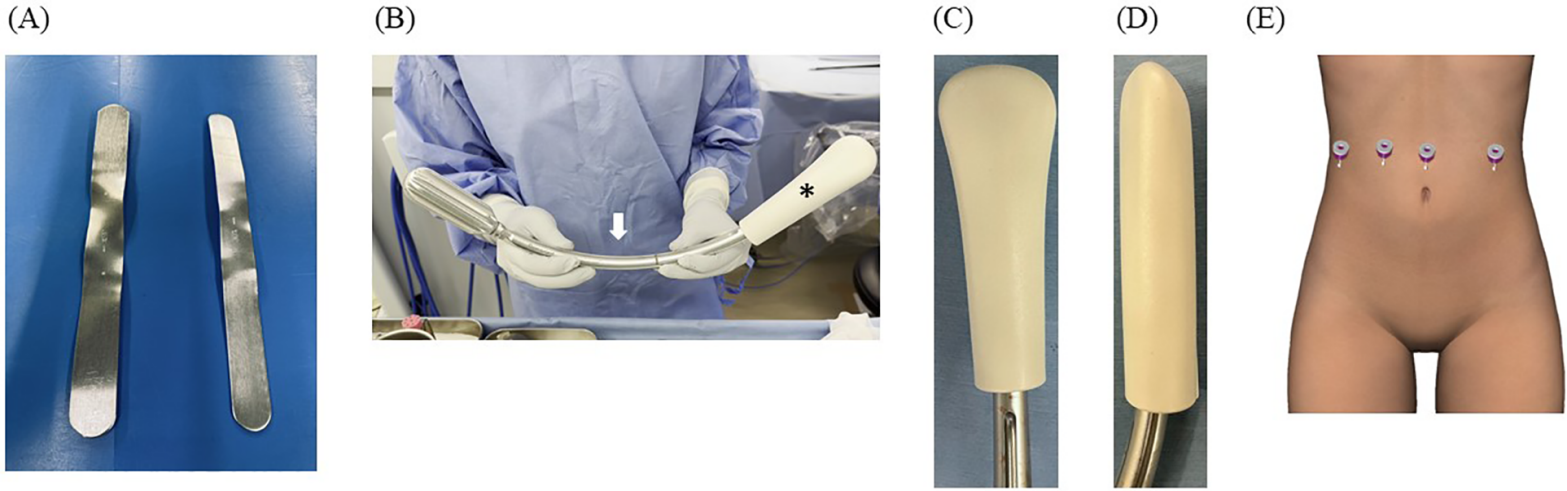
(A) The spatula on the left is 35 mm and on the right is 30 mm in width. (B) The vaginal manipulator (Hoyte Sacro Tip®, Cooper Surgical, Shelton, CT) consists of a reusable manipulator handle (white arrow) and a disposable sacrocolpopexy tip (black asterisk). (C and D) The Hyote Sacro Tip® is constructed in a uniquely thick dome shape. (E) Port placement for robotic-assisted sacrocolpopexy using the da Vinci Xi surgical system (Intuitive Surgical Inc., Sunnyvale, CA, USA).
